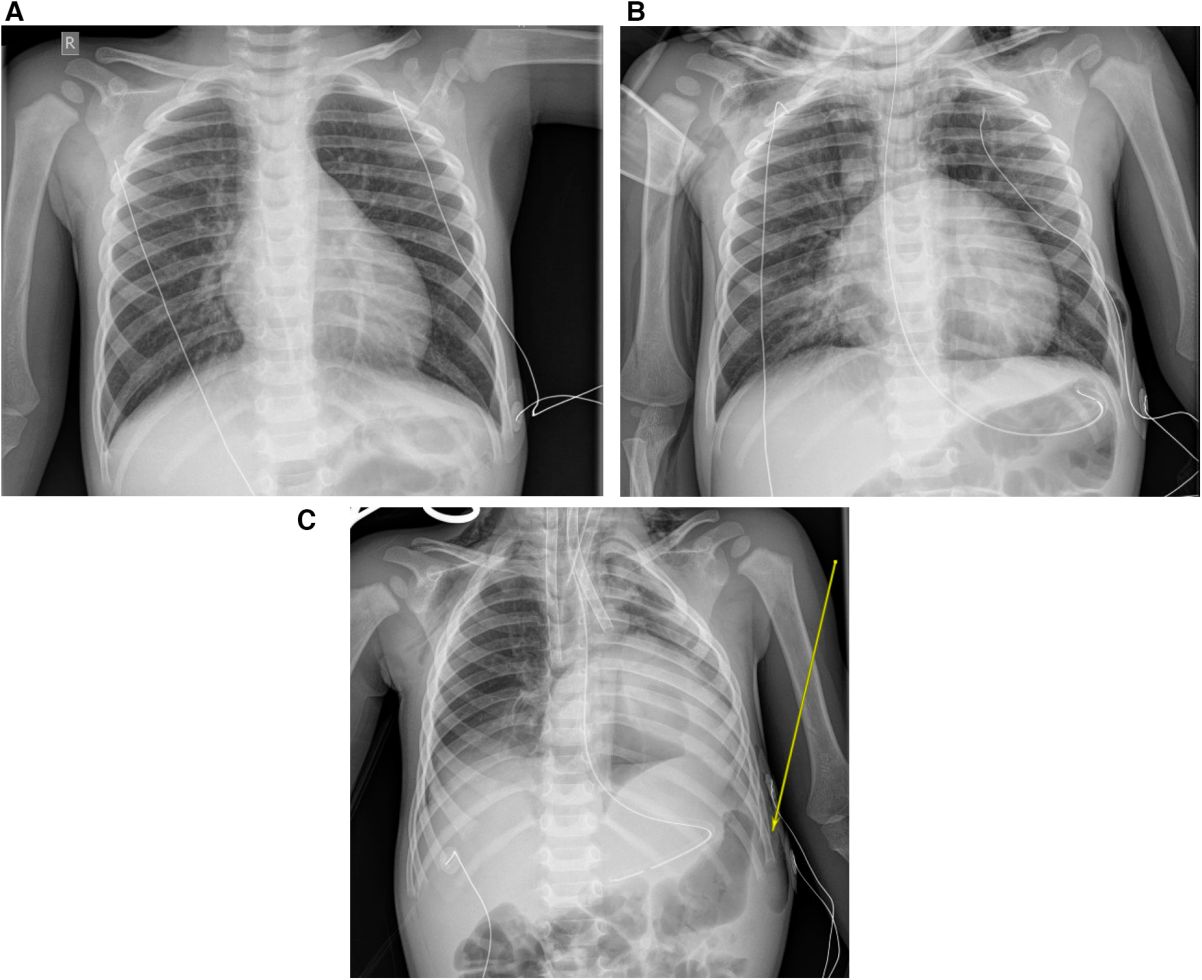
(A) Initial presentation chest x-ray. (B) Pre-intubation x-ray with complicated pneumomediastinum. (C) Post intubation and post-peripheral VA-ECMO cannulation.
